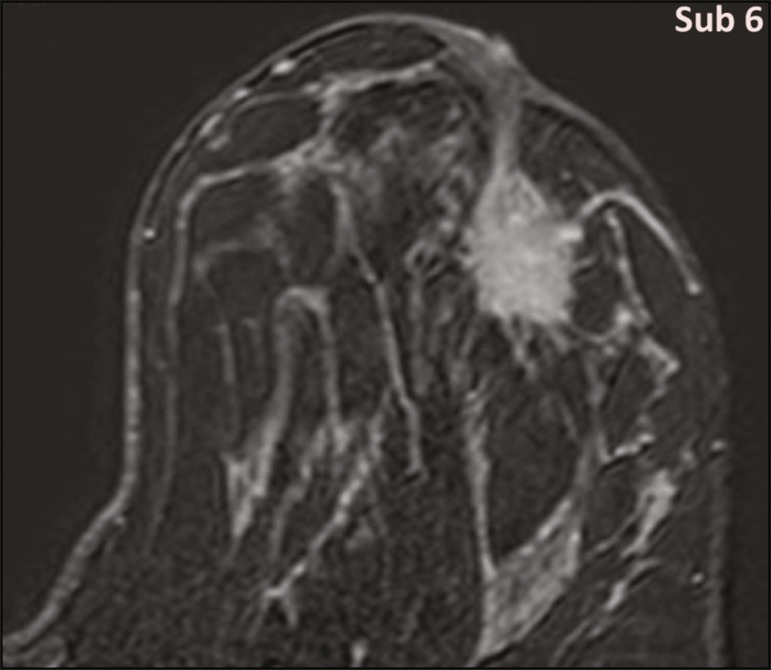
MRI of a 20-year-old woman (case 3) presenting with a mass in the upper outer quadrant of the left breast (delayed gadolinium enhancement—6 min—subtraction image). Note the skin retraction and spiculated margins, which suggest malignancy, although they essentially represent the desmoplastic reaction which is usually seen not only in carcinomas but also in GCT of the breast, as depicted in this pathologically confirmed GCT. The mass showed early mild enhancement with a type 1 kinetic curve.
